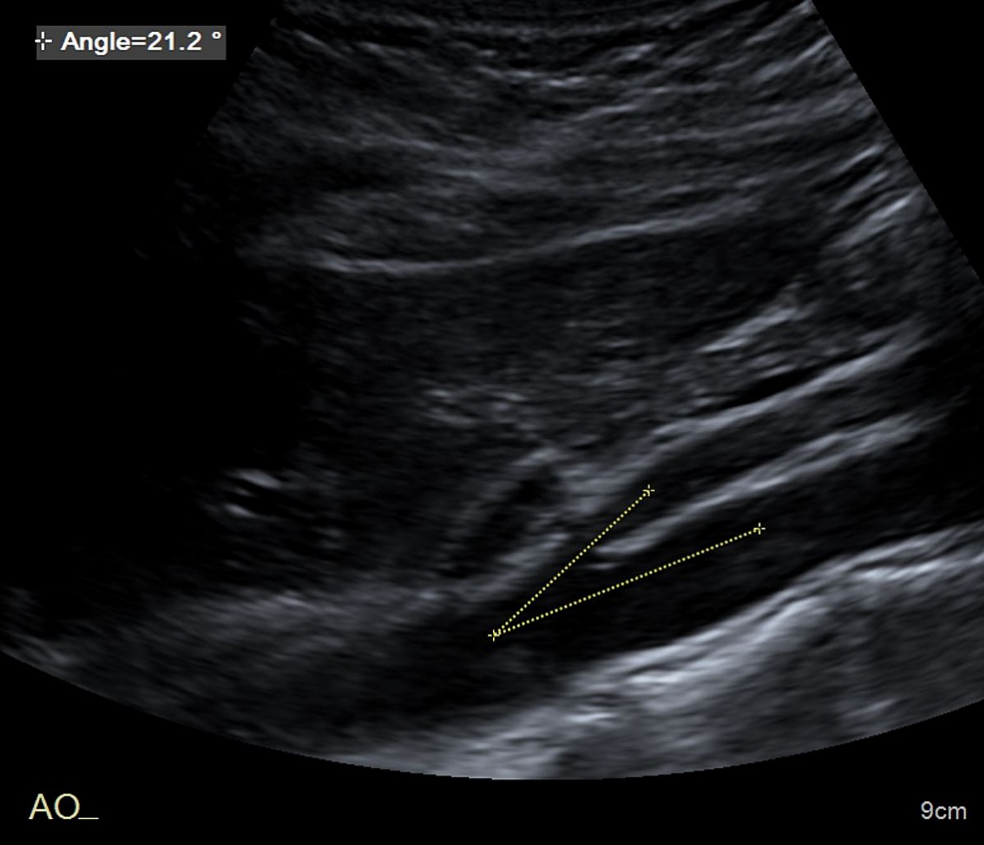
Sagittal abdominal ultrasound shows the angle between SMA and the aorta. SMA - superior mesenteric artery
